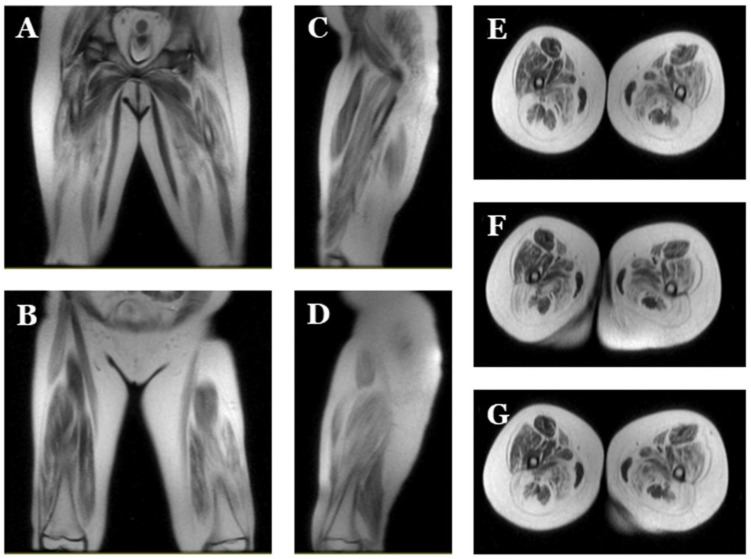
Magnetic resonance images of index case. Images of lower extremities showing diffuse bilateral atrophy with adipose infiltration. Coronal (A,B), sagittal (C,D) and axial (E–G) view.
